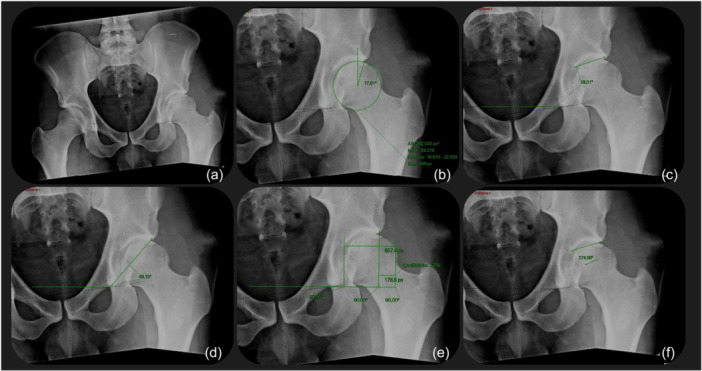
Adjusted radiographs. (a) Anteroposterior (AP) pelvis standard X‐ray. (b) Lateral centre‐edge angle (LCEA). (c) Acetabular index (AI). (d) Sharp angle (SA). (e) Extrusion index (EI). (f) Femoro‐epiphyseal acetabular roof (FEAR) index.
